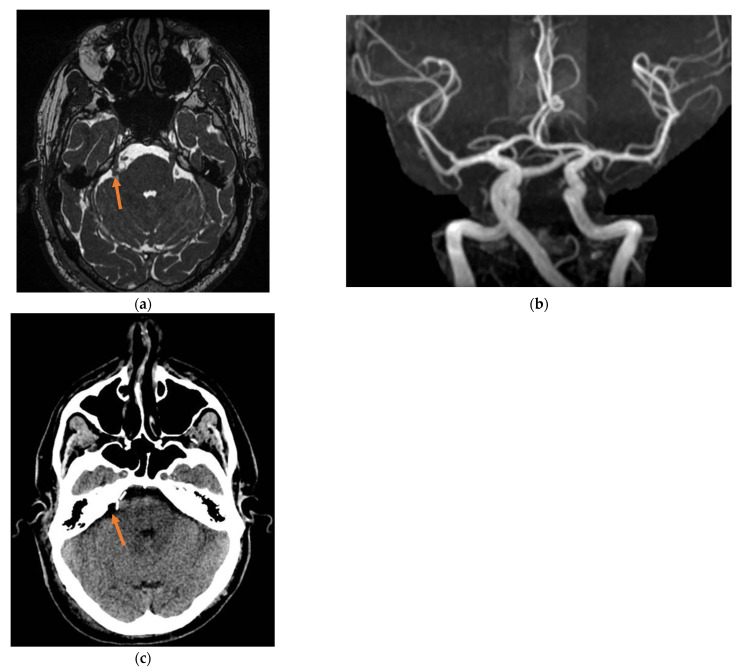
Post-surgery imaging showing Teflon sponges placed between the dolichoectatic basilar artery and the right trigeminal root (orange arrow). (a): MRI, axial plane, (b): MRI Angiogram, (c): CT scan, axial plane.
